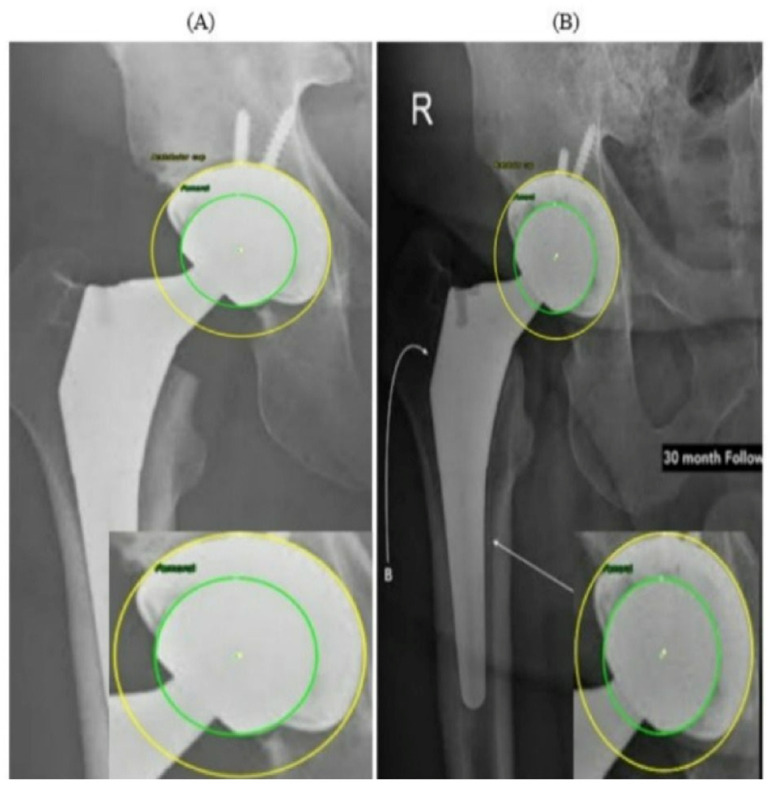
Example 1 of PRE–POST radiographs with detected femoral head and AC circles. (A) PRE radiograph demonstrating a centered femoral head within the acetabular cup. (B) POST radiograph showing superior displacement of the femoral head consistent with wear progression.
